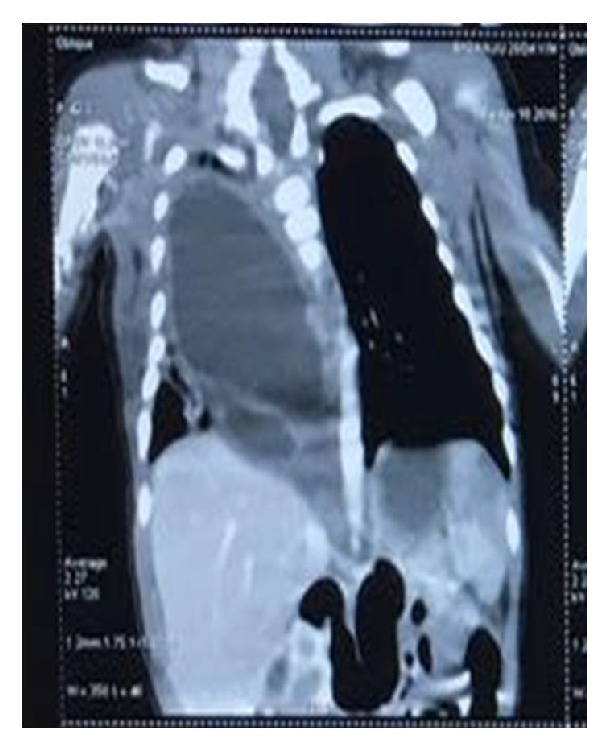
Computed tomography scan showed a well-defined fluid attenuation lesion with broad base towards the mediastinum with few loculations.
